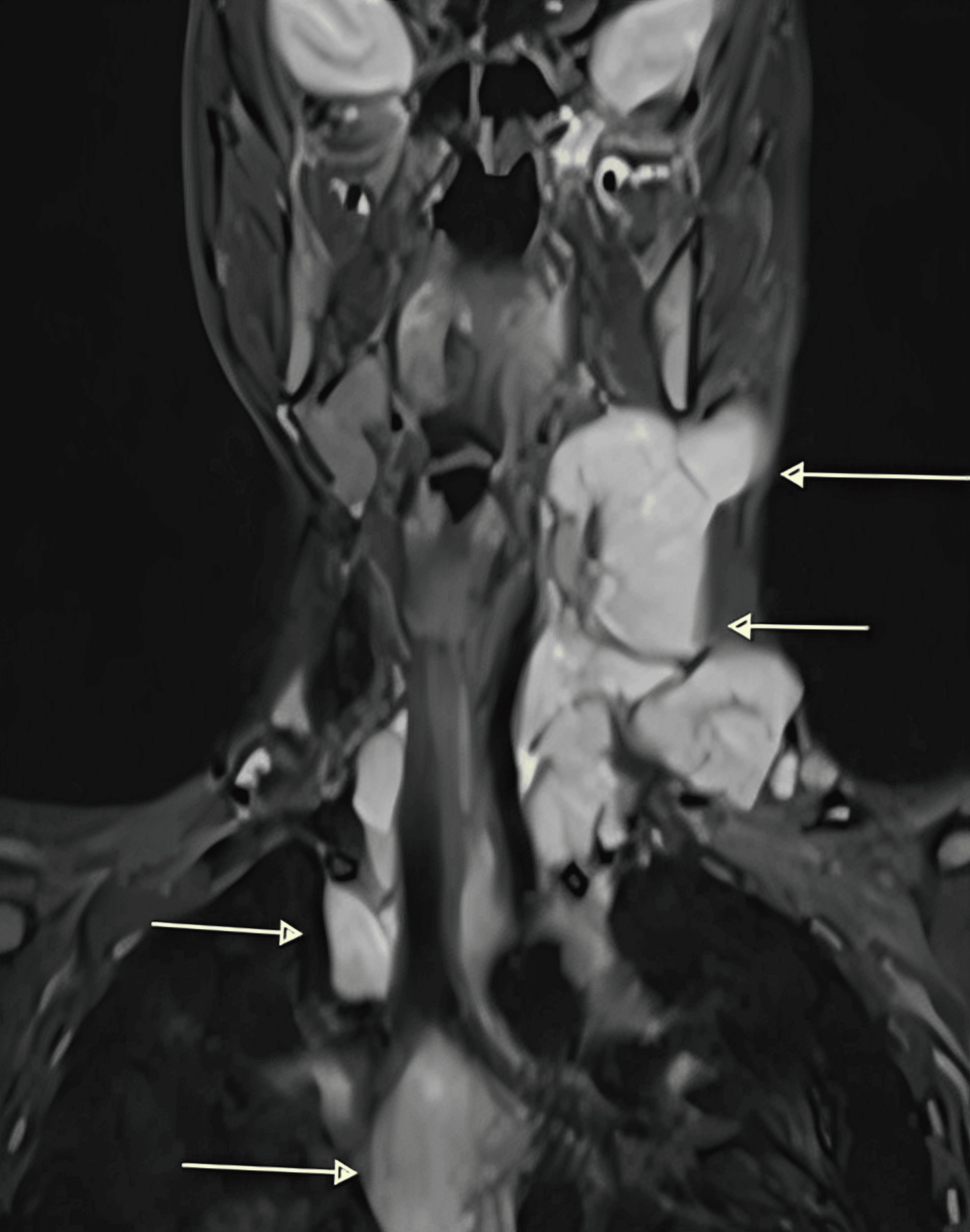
Contrast-enhanced computed tomography (CECT) of the neck showing multiple enlarged, homogeneously enhancing lymph nodes (arrow marked)
